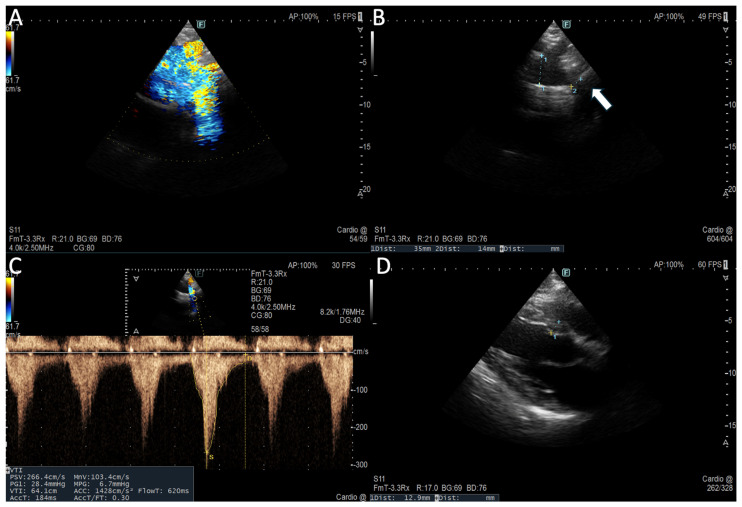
Assessment of aortic coarctation by TTE. (A) Aortic arch colour imaging with turbulent flow in suprasternal window. (B) Narrowing of isthmus diameter (with arrow) compared to proximal aortic arch (ratio 0.4) in suprasternal window for anatomical assessment. (C) Continuous doppler showing peak systolic velocity (>2 m/s) and peak pressure gradient (>20 mmHg) across narrowing site for functional assessment of significant CoA gradient. (D) Indirect CoA finding of LV hypertrophic remodelling.
